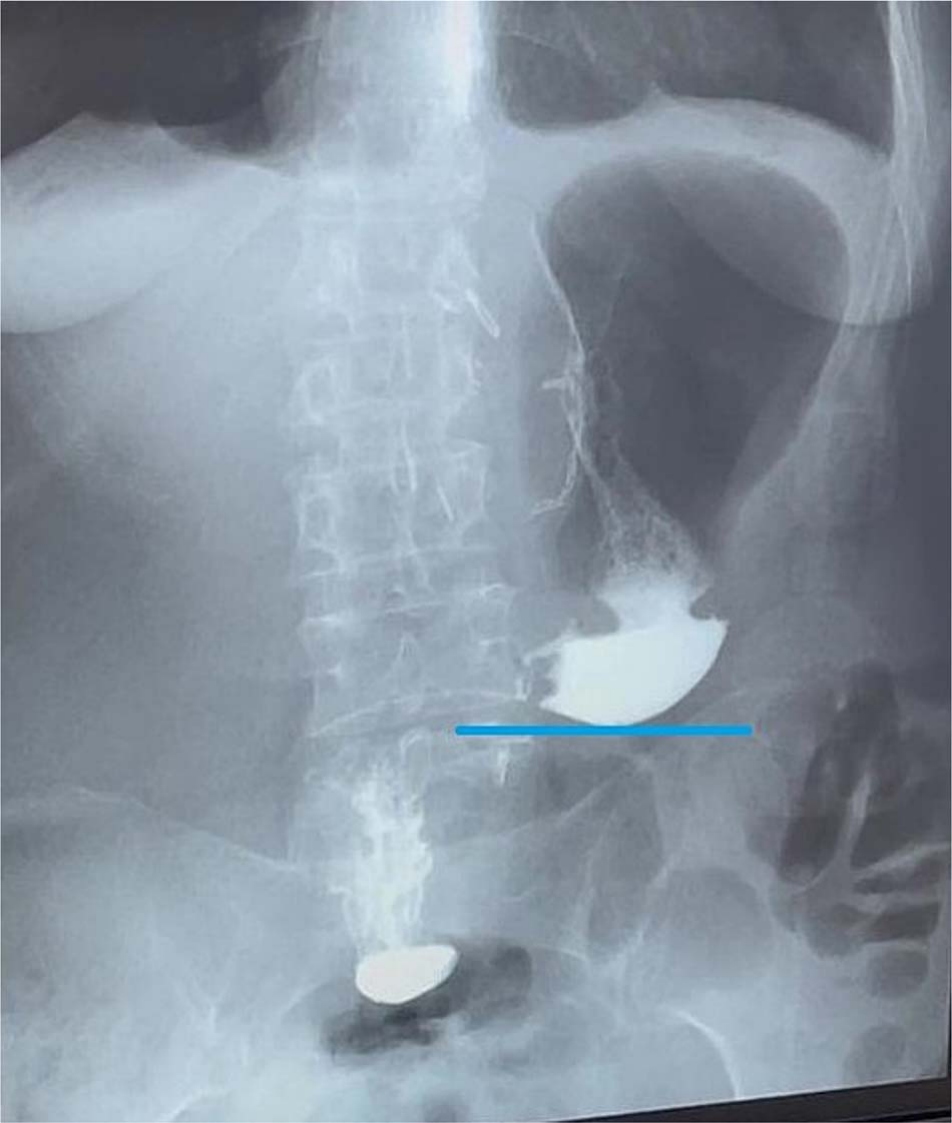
Contrast X-ray study performed on the third postoperative day. The image demonstrates free passage of contrast through a widely patent gastroduodenal (Billroth I) anastomosis without evidence of leakage or obstruction. Line indicates the newly configured lower border of the gastric remnant.
